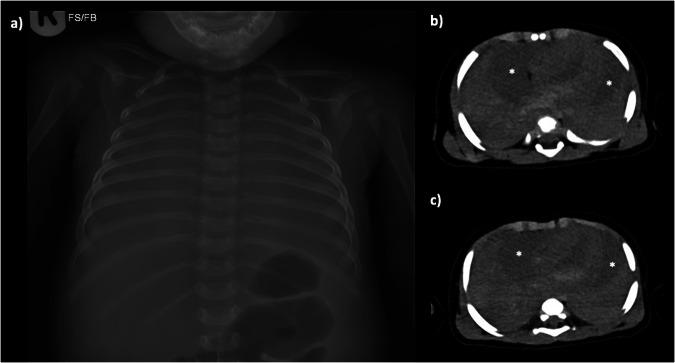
Postmortem chest radiograph (a) and axial PMCT images through the thorax (b, c) in a 5-month-old girl found unresponsive. The PMCT demonstrates a large pericardial effusion (asterisks) with cardiomegaly, not visible on chest radiography. In the absence of a history of peri-resuscitative or postmortem needle sampling of this fluid, it should be presumed to be an antemortem. The dense consolidated appearances of the lungs were considered normal postmortem change
